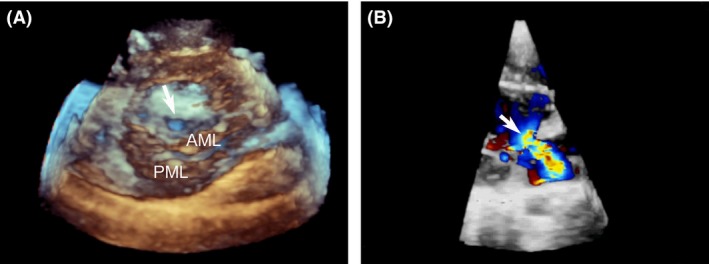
Real‐time three‐dimensional transthoracic echocardiography revealed a hole in the A2 region (arrow) near to the anterior annulus of the anterior mitral leaflet (AML). Real‐time three‐dimensional color flow full volume imaging showed severe MR originated from anterior mitral leaflet perforation (arrow). PML, posterior mitral leaflet.
